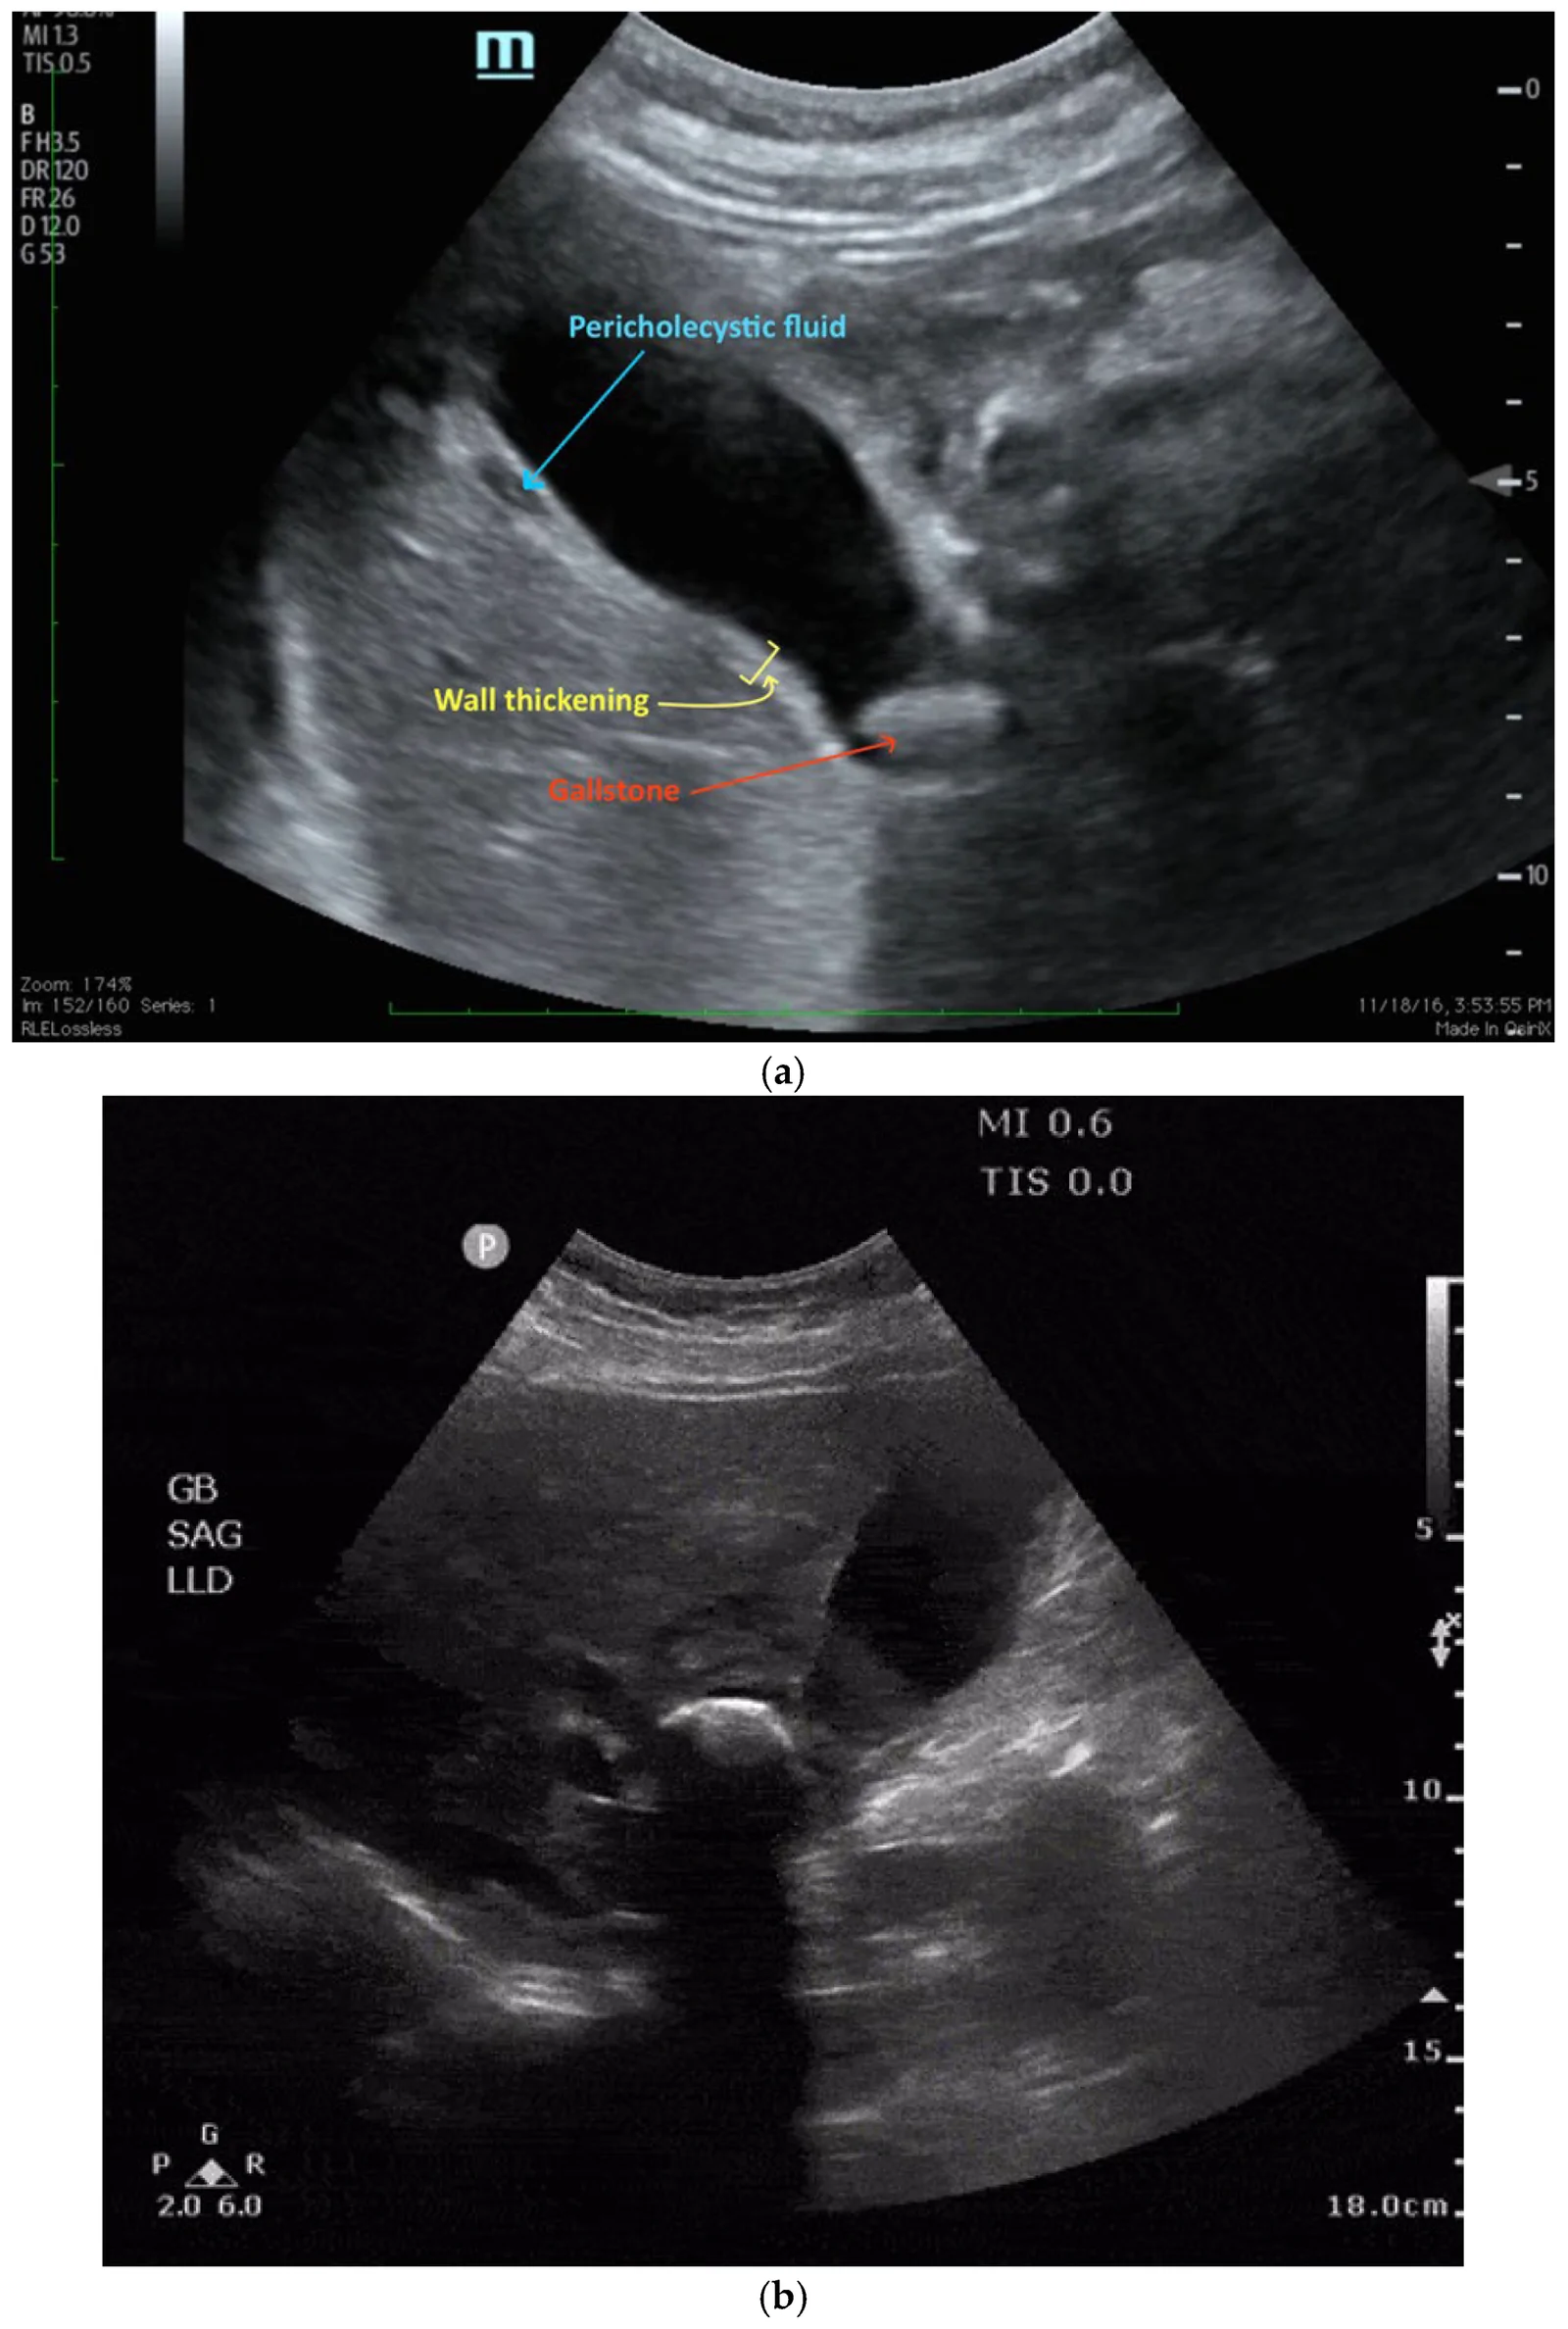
(a,b) Obstruction of the cystic duct by a gallstone can cause gallbladder inflammation and can develop into acute cholecystitis. Cholelithiasis causing obstruction at the gallbladder neck is the most common cause of acute cholecystitis, which accounts for up to 9% of hospital admissions for abdominal pain [7]. For detection of acute cholecystitis, POCUS accuracy varies across studies and has ranges reported as 71–88% sensitivity and 80–100% specificity [3,4,8,9,10,11]. Moreover, a systematic review and meta-analysis performed in 2024 across 7 databases compared emergency physicians performing POCUS against a reference standard of final diagnosis (surgical pathology, discharge diagnosis, and radiology-performed ultrasound). They found a pooled sensitivity of 70.9% (95% confidence interval [CI] 62.3 to 78.2), specificity of 94.4% (95% CI 88.2 to 97.5), positive likelihood ratio of 12.7 (5.8 to 27.5), and negative likelihood ratio of 0.31 (0.23 to 0.41) for the diagnosis of acute cholecystitis [7]. Based on this performance data, POCUS is useful for detecting disease, but a negative or technically limited scan does not exclude acute cholecystitis when clinical suspicion remains high. Key identifying factors on ultrasound include gallbladder wall thickening greater than 3 mm (measured anteriorly), presence of pericholecystic fluid, and a positive sonographic Murphy’s sign. This is defined as pain elicited with probe pressure over the gallbladder and lack of pain with the probe at another site [3,4,9,10]. (a) is an example of an obstructing calculus causing gallbladder inflammation with pericholecystic fluid and gallbladder wall thickening. (b) is an example of a stone in the gallbladder neck that is causing external compression of the biliary tree, which is a less common disease known as Mirizzi syndrome. Prolonged stone immobility can cause chronic inflammation and fibrosis [3,4]. In cases that are equivocal for acute cholecystitis (only meets 1–2 of the above diagnostic imaging criteria) or symptomatic cholelithiasis, surgical consultants may request additional imaging. This could be a RUQ radiology ultrasound, CT scan, or a hepatobiliary iminodiacetic acid (HIDA) scan to evaluate for complete gallstone obstruction of the cystic duct for emergent surgical planning. A HIDA scan is appropriate for patients with high clinical suspicion of cholecystitis but equivocal ultrasound findings [12]. With acute cholecystitis, the HIDA scan will show no nuclear tracer uptake into the gallbladder within 1–4 h due to an obstructed cystic duct. Whereas, CT scan is better for complicated or post-operative patients with altered anatomy [12]. In a study by Oda et al. comparing CT scan and ultrasound for diagnosis of acute cholecystitis, CT was recommended as a follow up study for equivocal cases with high sensitivity 85% and specificity 100% [10].
